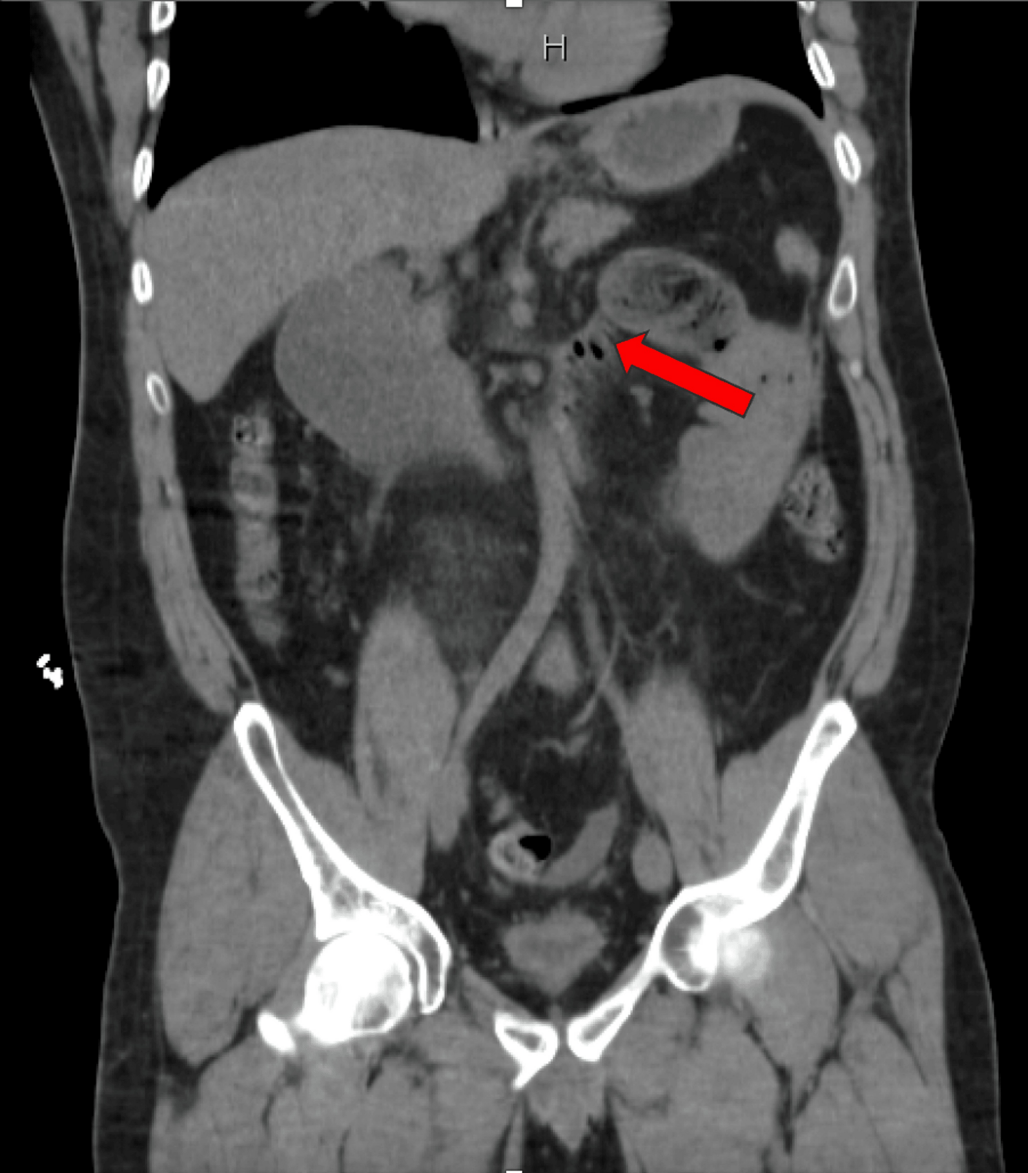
CT scan of abdomen and pelvis showing transition point in the left upper quadrant (denoted by the red arrow)
